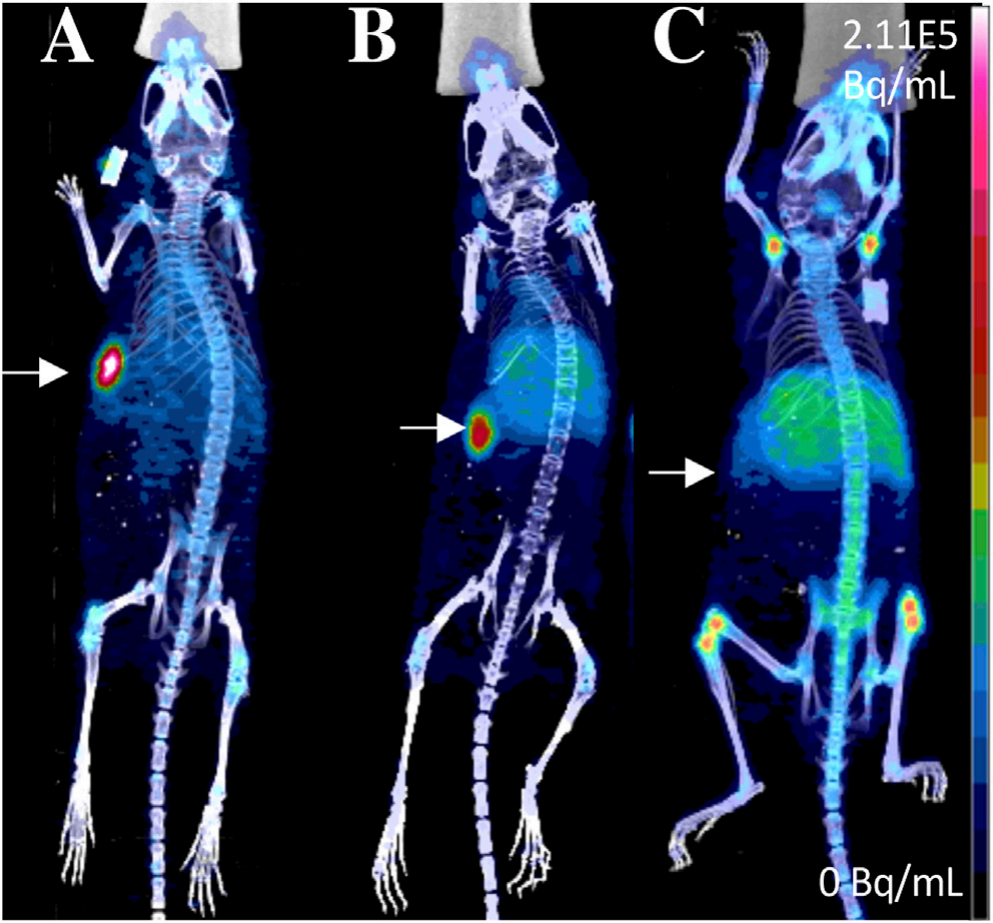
PET/CT imaging of [^89^Zr]ZrDFO-Amivantamab in MDA-MB-468 (A), MDA-MB-231 (B) and MDA-MB-453 (C) xenografts of TNBC at 96 h p. i. Tumors are marked with arrows (Cavaliere et al., 2020). The general structure of [^89^Zr]ZrDFO-Amivantamab was shown in Figure 2H.
